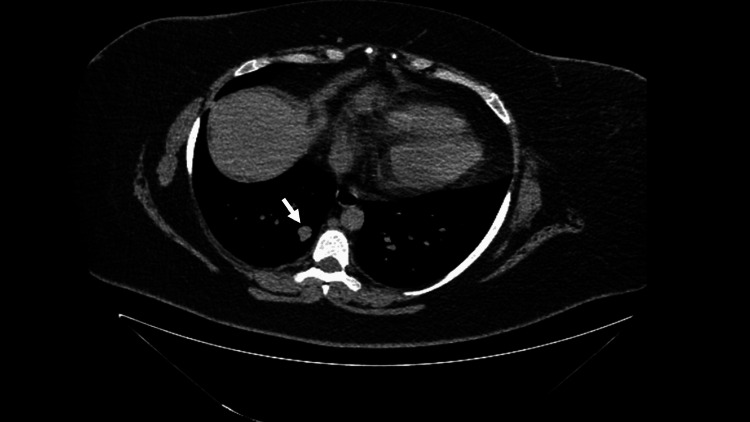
Computed tomography (CT) of the chest showed a right 1.2 cm lung nodule (white arrow).
